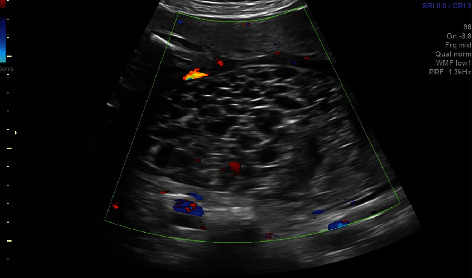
Close of color Doppler of molar placenta.
